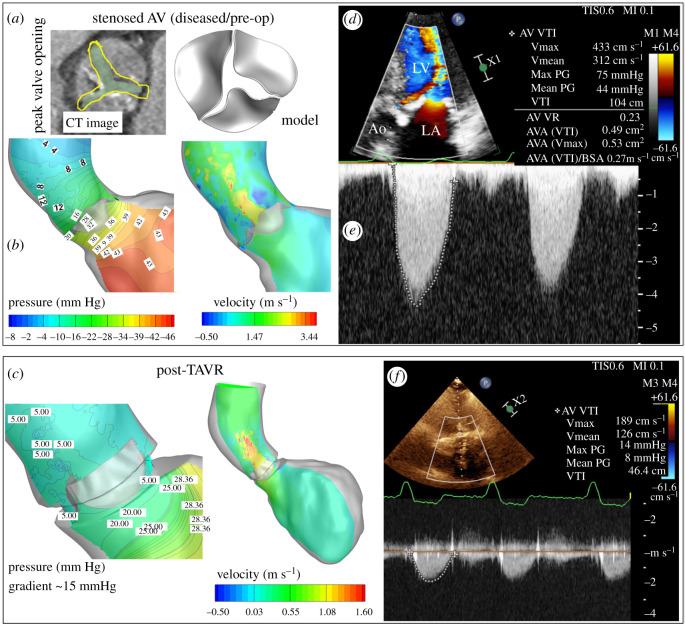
Clinical validation of model predictions with pre- and post-TAVR. (*a*) Comparison of maximum orifice from CT and model prediction for SAV; (*b* and *c*) model predictions of transvalvular pressure gradient and velocity pre- (SAV) and post-TAVR respectively, have a good agreement with (*d*–*f*) corresponding Doppler ultrasound data (three-chamber view) measured pre- and post-TAVR.
